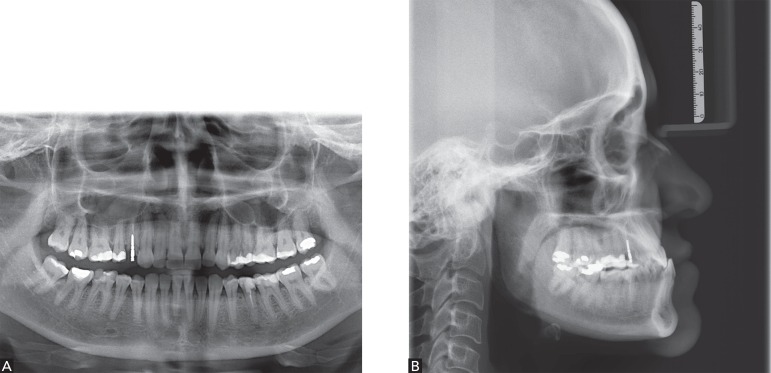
Initial photographs: A) Panoramic radiograph; B) Profile
